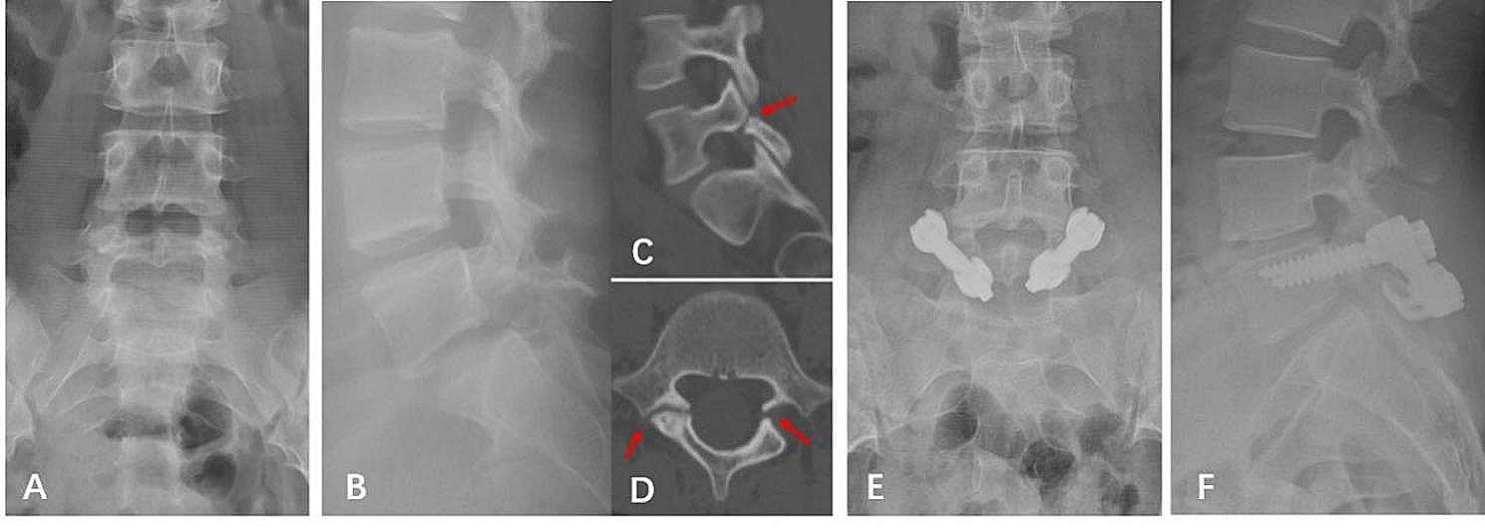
Preoperative lumbar spine X-rays of a 15-year-old female diagnosed with L5 spondylolysis (A, B). Sagittal and axial CT images showing the defects in the pars interarticular (C, D). Postoperative lumbar spine x-rays showing the pedicle screw- rod-laminar hook system (E, F)
